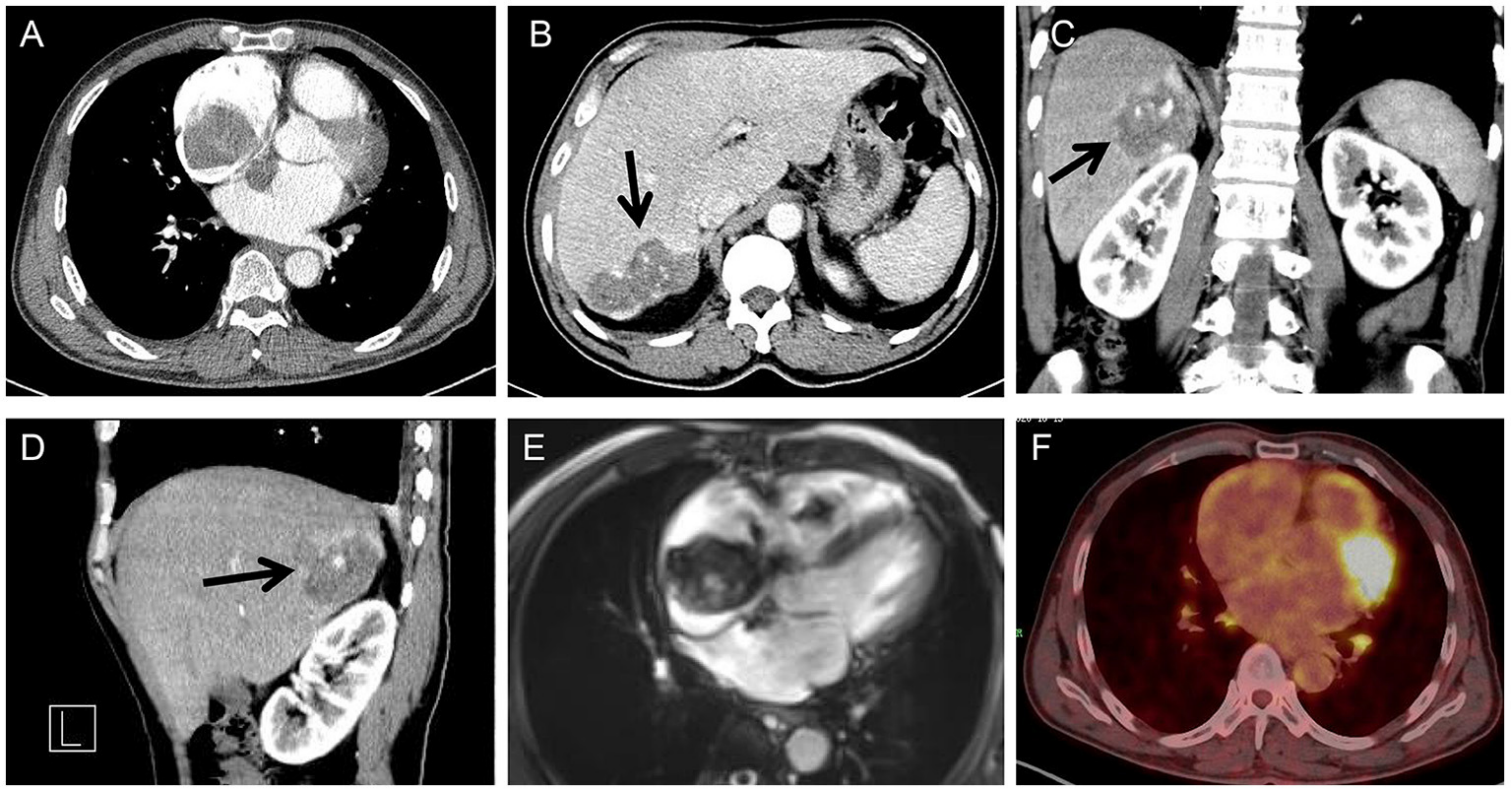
Cardiac CT showing a biatrial mass with the atrial septum infiltrated (A); Abdominal CT showing an angiomatous mass in the right posterior lobe of the liver (arrow) (transverse view) (B), coronal (C), and sagittal (D) plane; cardiac MRI showing a giant biatrial mass with tricuspid valve involved (E); PET-CT showing a giant mass with increased glucose metabolism in the right atrium (F).
